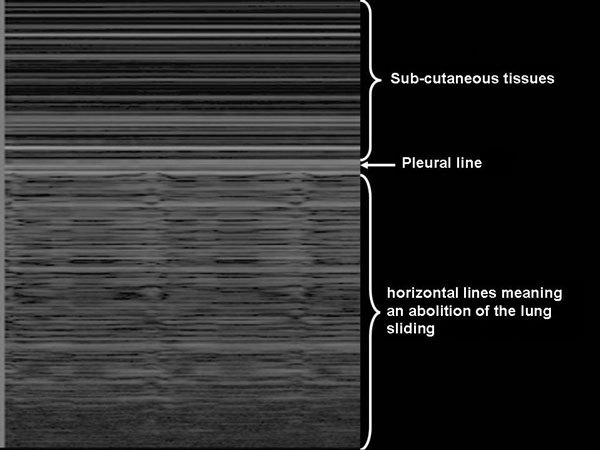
Abolition of lung sliding on pleural ultrasonography in time-motion mode in a patient with pneumothorax [31]. While the patient is breathing, the (normal) granular pattern under the pleural line is replaced by horizontal lines, indicating abolition of lung sliding. Reproduced with permission (ACCP - Chest).
